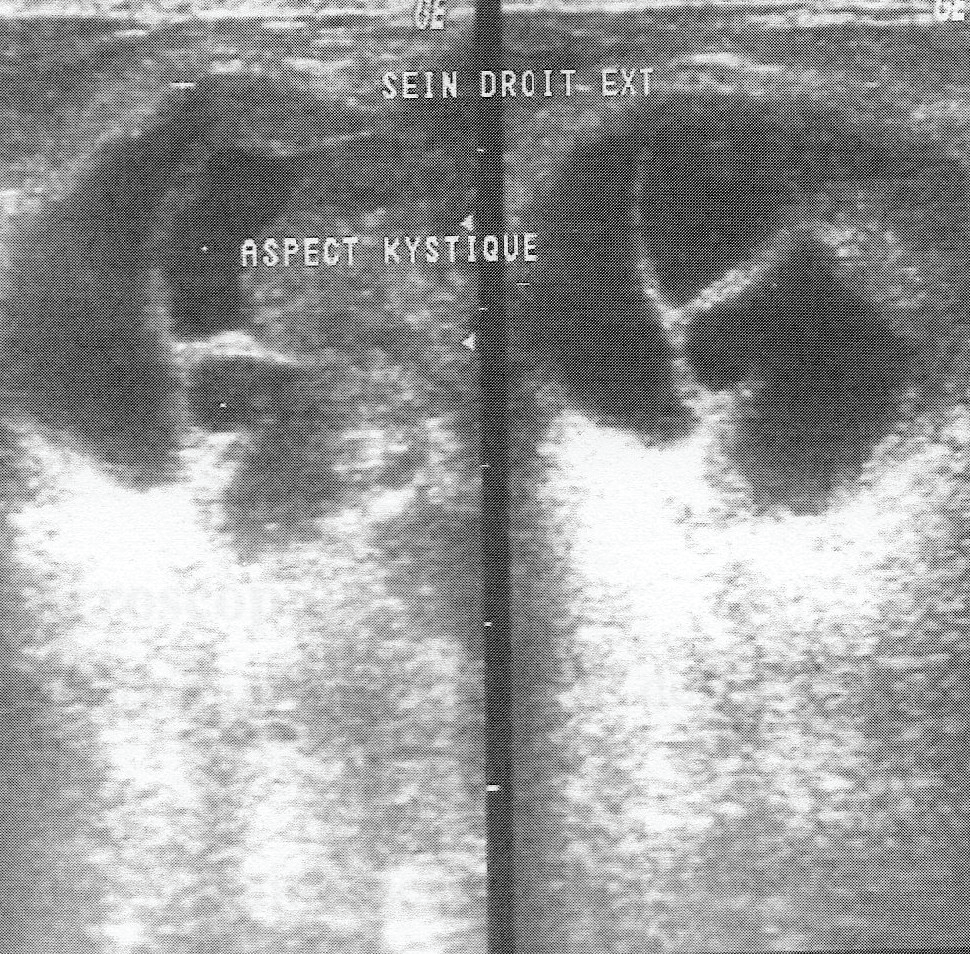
Ultrasound of the breast revealing a solid smooth bordered mass with cystic spaces.
